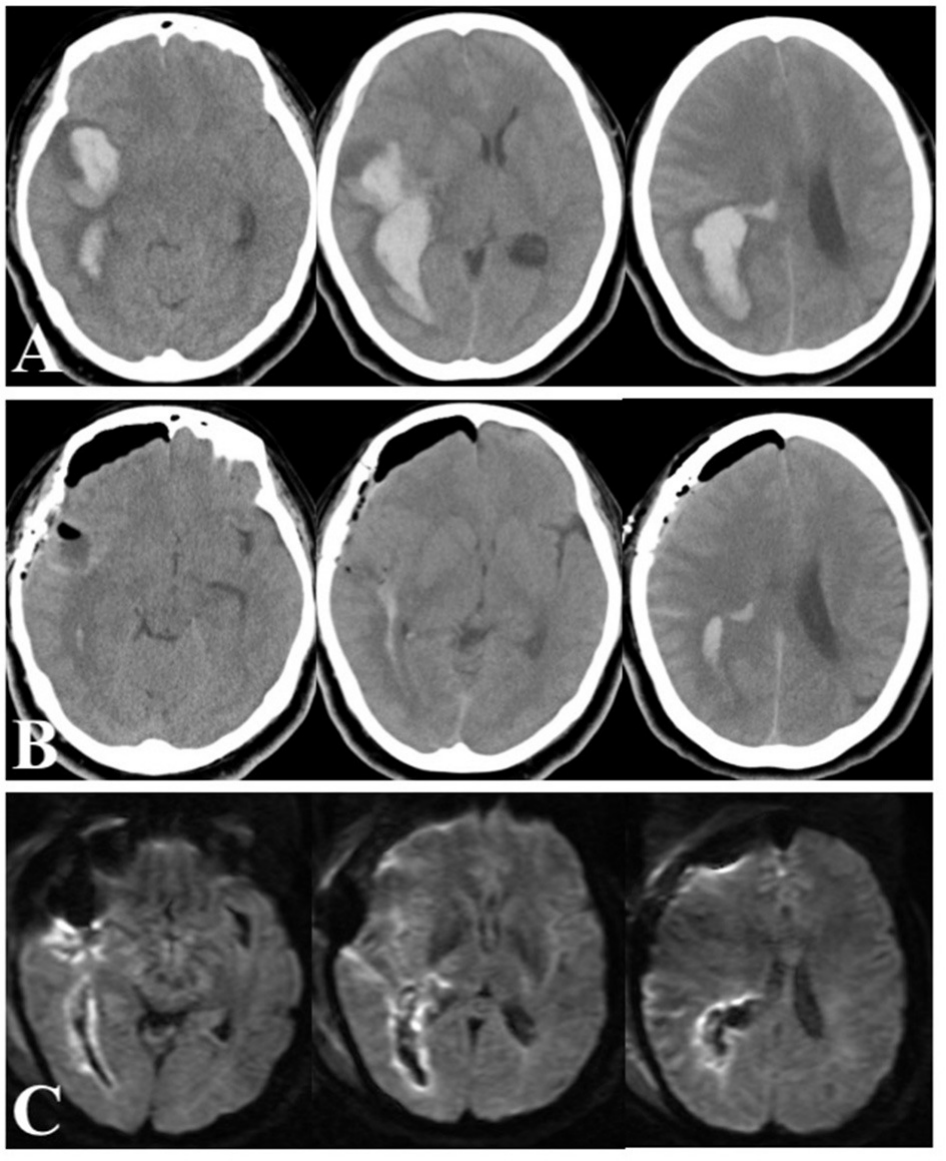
Head CT scans on arrival (A), immediately after ICH removal (B), and diffusion-weighted MRI images next day (C).
